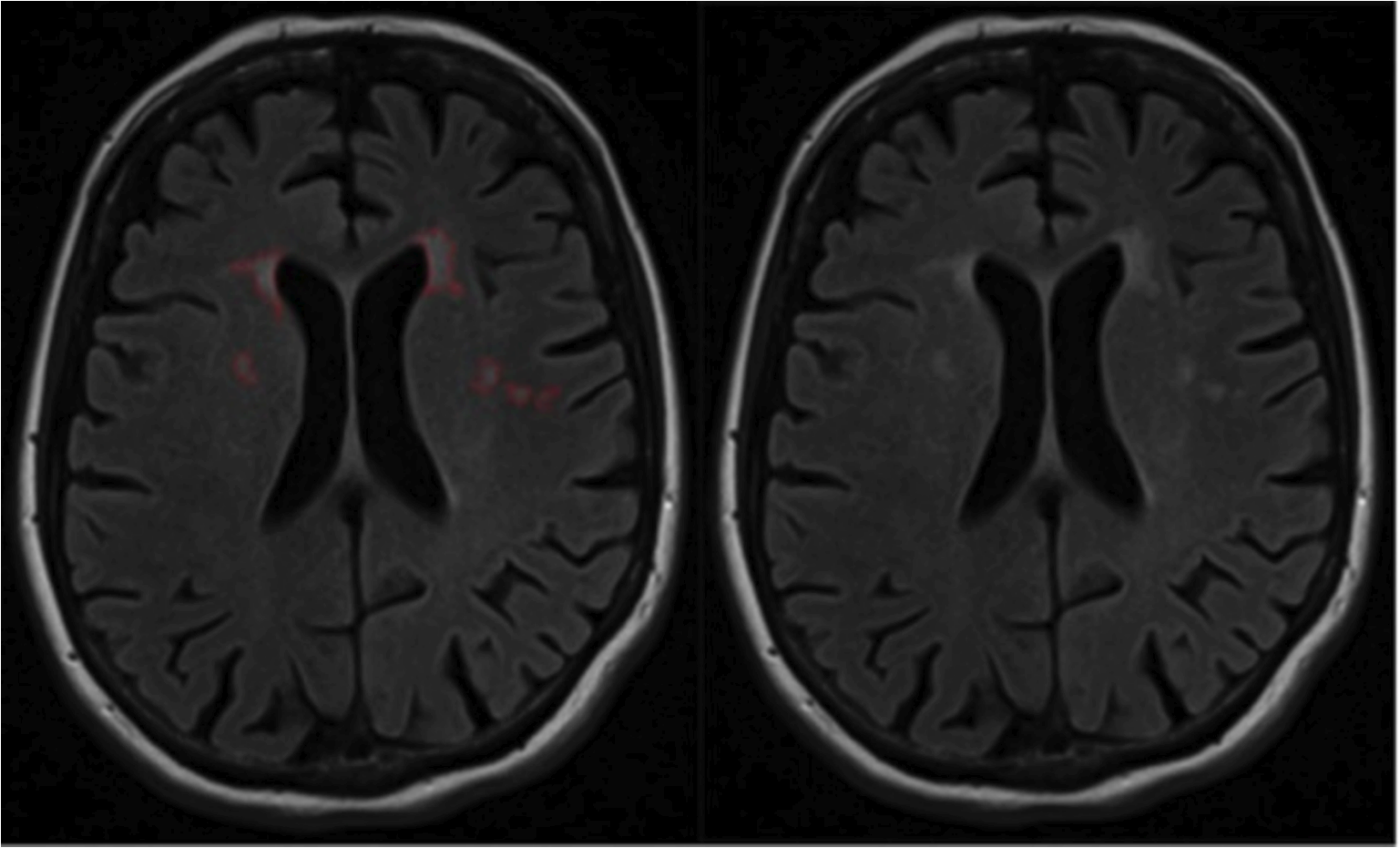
Representative FLAIR slice of one patient. The left image shows an example of lesion areas, outlined semiautomatically by the instructed rater using a custom written IDL program (Exelis Visual Information Solutions, USA). The right image shows the native FLAIR scan.
